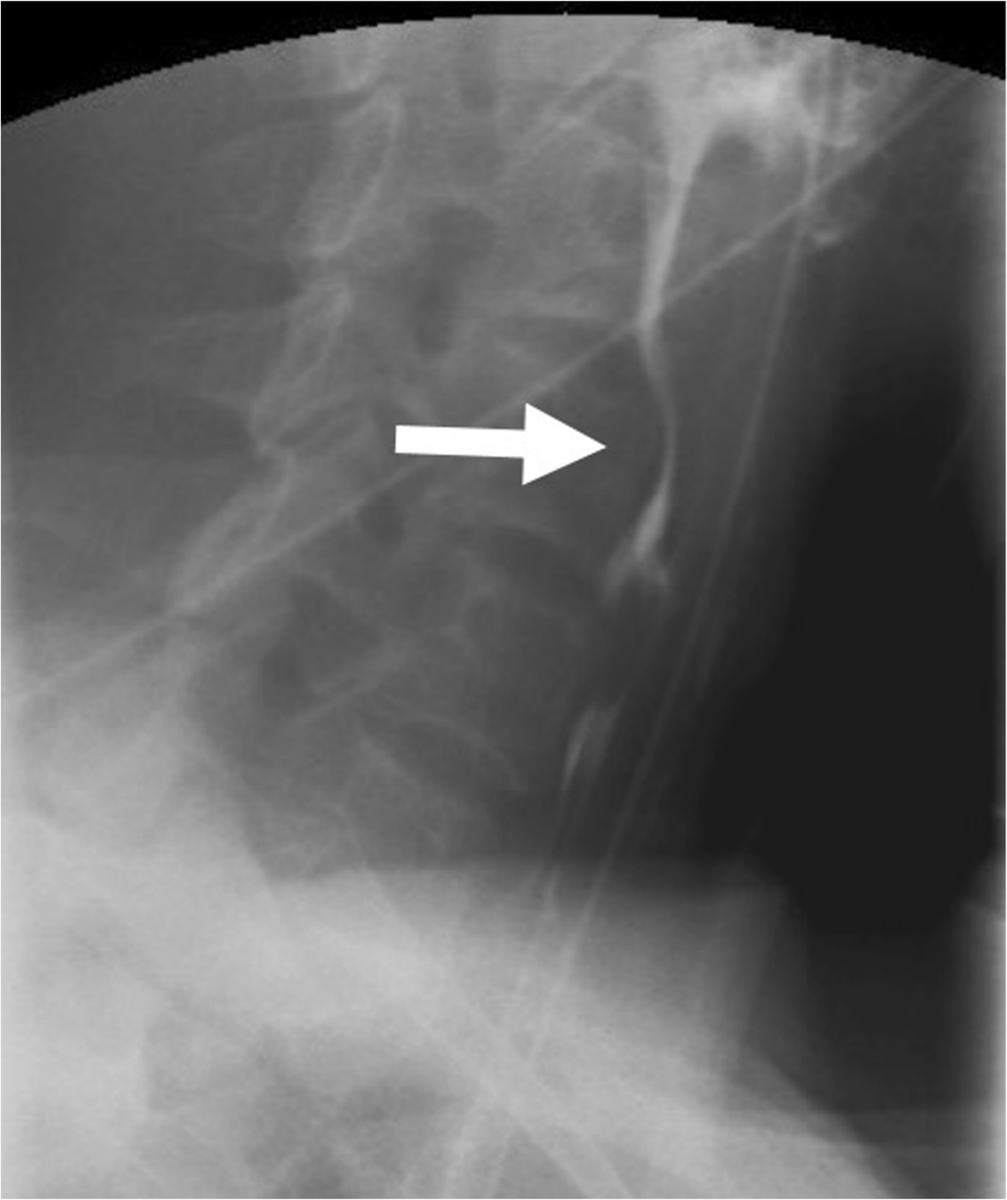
A Cricopharyngeal narrowing was seen in the lateral projected barium radiographic study*
